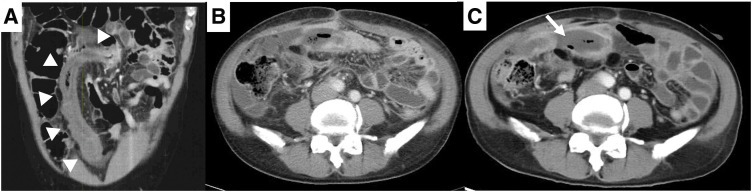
(A) CT at the initial examination at the previous hospital. The patient had wall thickening from the rectum to the sigmoid colon (white arrowhead). (B) CT at the last examination after colostomy at the previous hospital. Enteritis had worsened, and ascites had increased. (C) CT before colectomy at our hospital. There was edematous wall thickening from the sigmoid colon to the rectum, with partial wall disruption in the sigmoid colon. Peritoneal fluid accumulation with encapsulation (white arrow), peritoneal thickening, and increased density of abdominal adipose tissue were observed.
